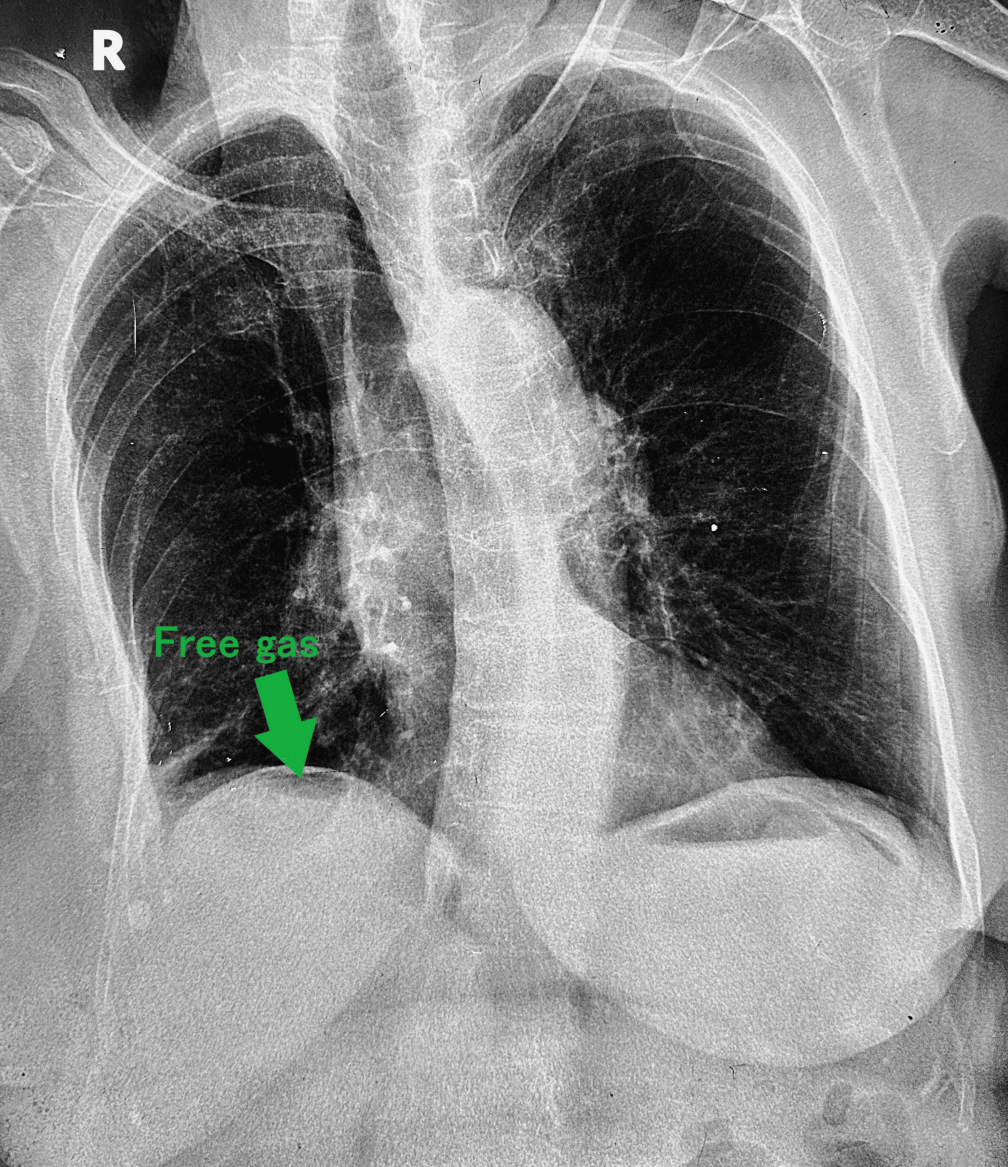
Erect chest X-ray demonstrating pneumoperitoneum. Erect chest X-ray showing subdiaphragmatic free air (free gas, marked by an arrow), consistent with pneumoperitoneum. This finding suggests the presence of intra-abdominal perforation, necessitating urgent clinical evaluation.
